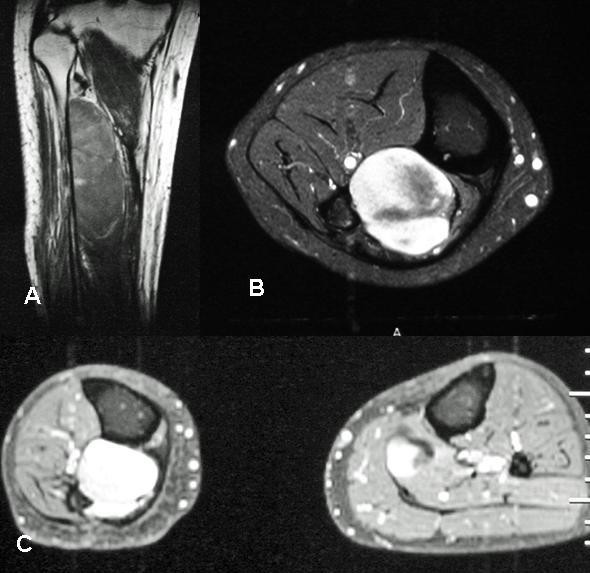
A& B – MR image (longitudinal & transverse) of the right leg showing a large myxoid liposarcoma (Case 3). C) – MR Transverse sections of both legs (Case 3) showing a secondary lesion in the soleus muscle on the left side along with an aggressive recurrence of the primary on the right side.
